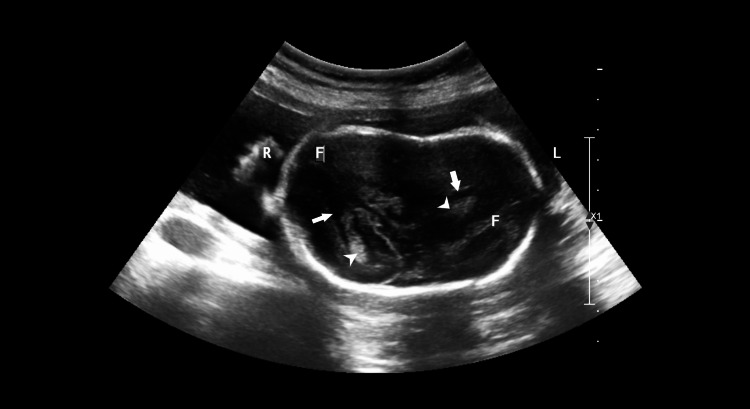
Transverse ultrasound image showing two fused skulls with normal bone ossification. Two separate brains with separate falx cerebri (F) are noted. The image also shows bodies of lateral ventricles (arrows) containing choroid plexus (arrowheads).
